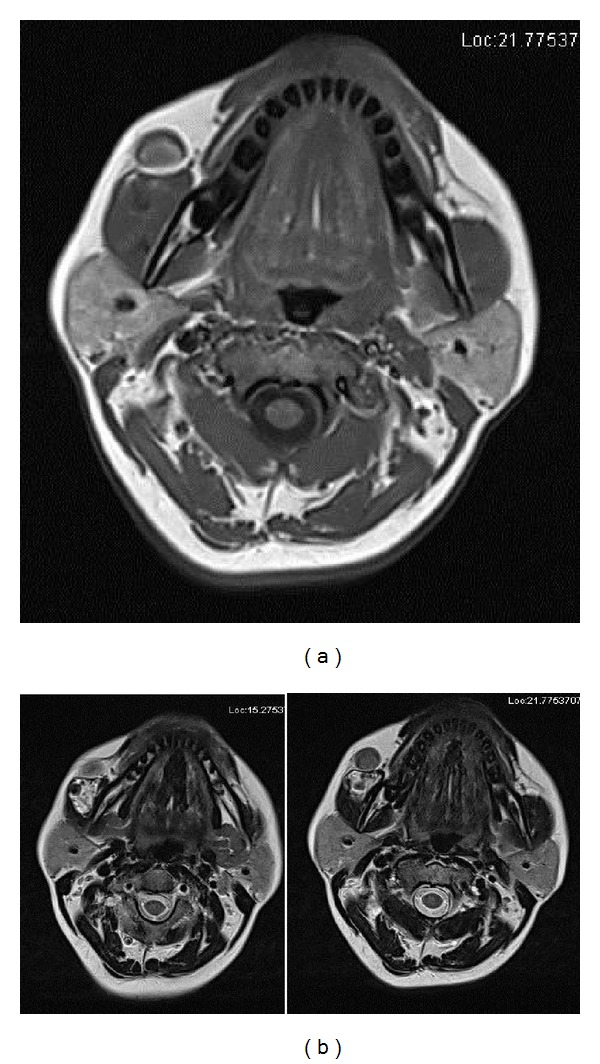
(a) Magnetic resonance imaging discloses isointense mass on T1-weighted MR image. (b) Isointense mass on T2-weighted MRI.
